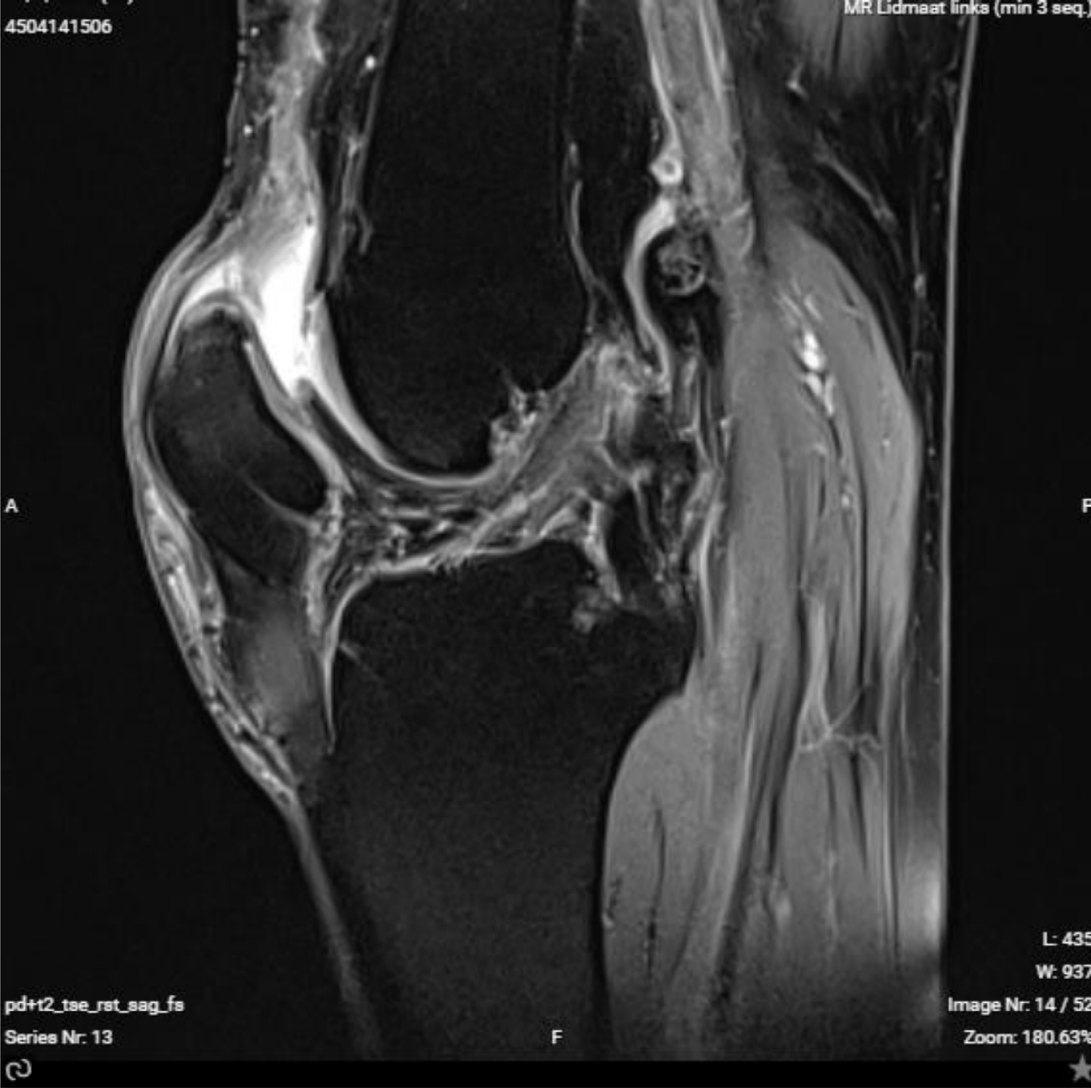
MRI showing the quadriceps lesion.
